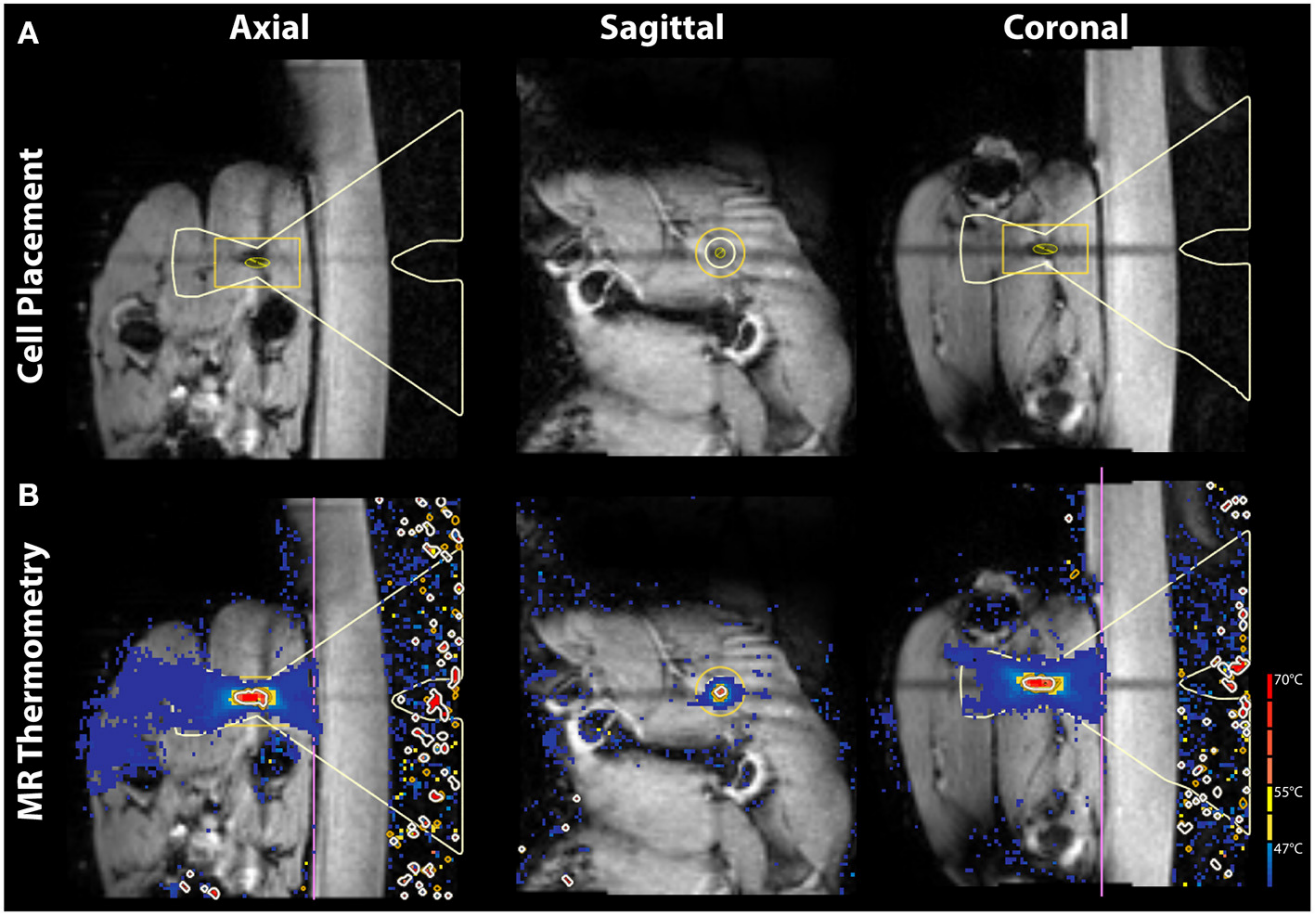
Pre-treatment planning images and in-treatment MR thermometry in axial, sagittal, and coronal view. (A) T1-weighted images are shown with the MRgFUS treatment cell placed on the sciatic nerve. (B)
